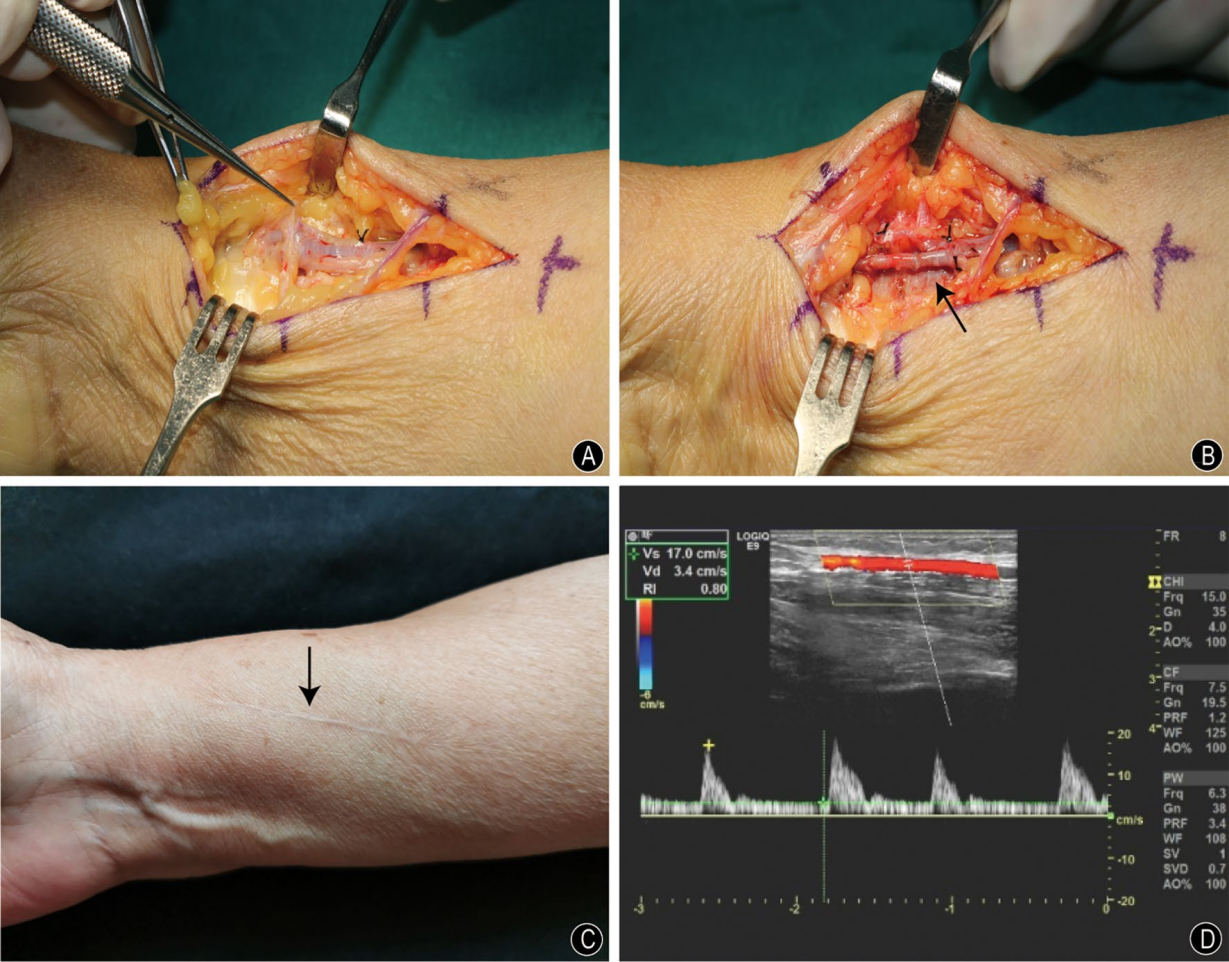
Intraoperative and postoperative pictures. A The artery and the vein within the fistula were mixed up. B After resecting and ligating the fistula, the identified radial artery was anastomosed (arrow). C The photo showed that the incision was well healed, with no swelling and thrill (arrow). D Doppler ultrasound showed the radial artery remained intact, with antegrade flow preserved
